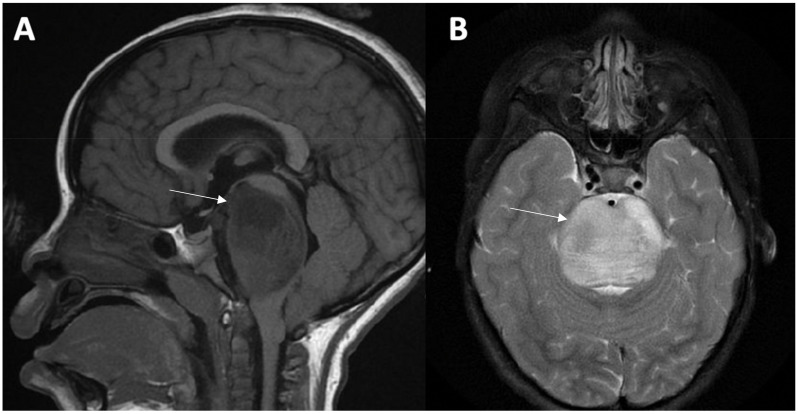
DIPG Imaging Findings. MRI of the brain with and without gadolinium in a child with an *H3 K27*-altered, DIPG. The imaging demonstrates a sagittal T1 hypointense (A) and axial T2 hyperintense (B) lesion with homogeneous enhancement along with obstruction within the fourth ventricle of the brain occupying almost half of the axial diameter.
